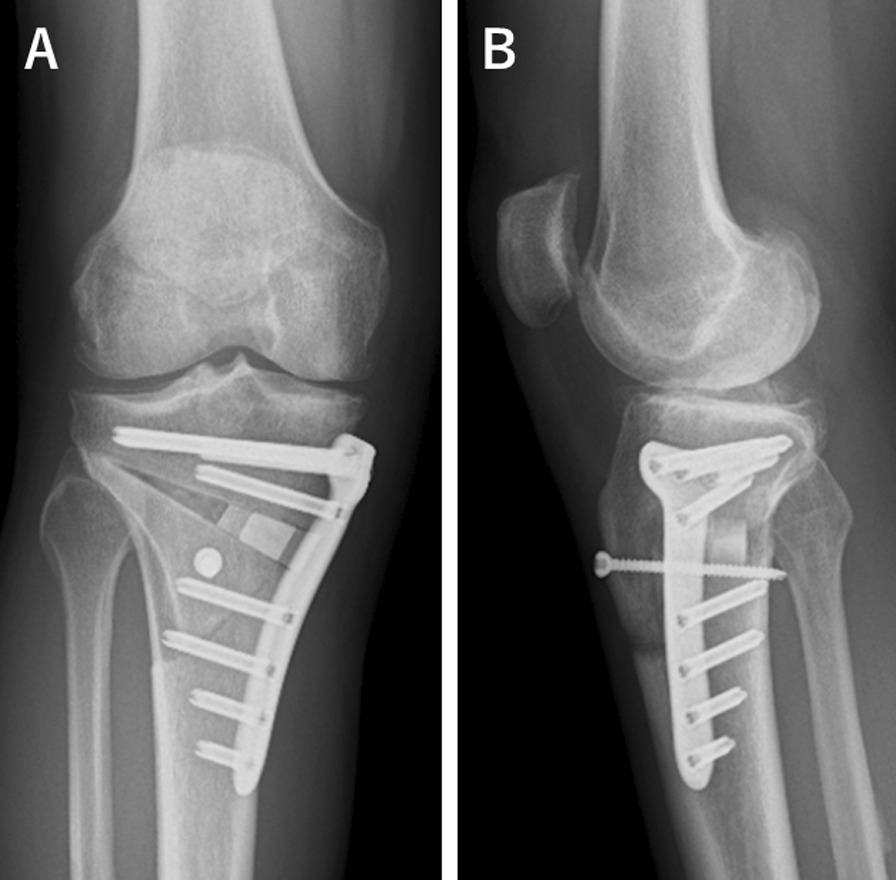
Postoperative radiograph of the right knee. A anteroposterior view. B lateral view
